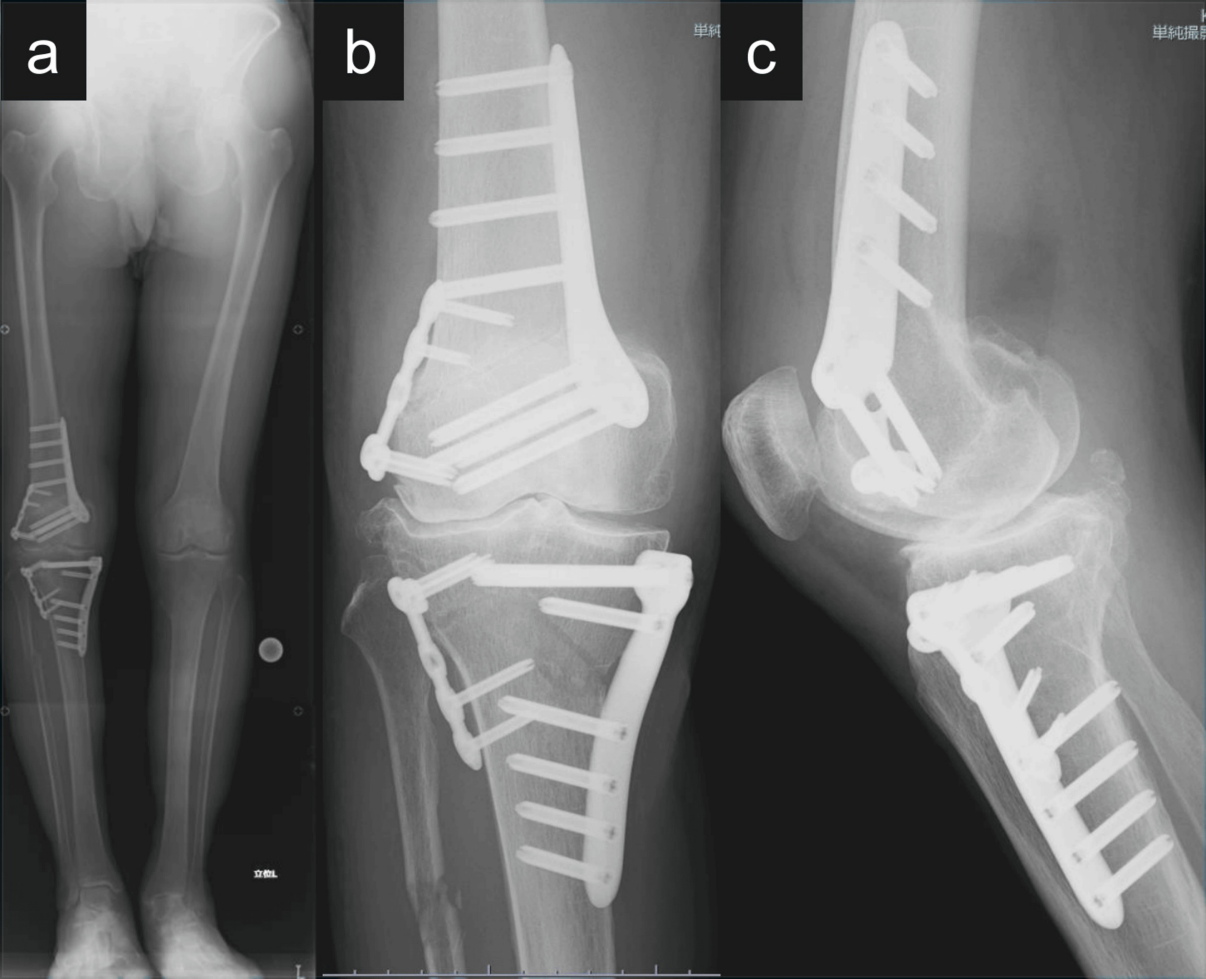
Immediate postoperative radiographs of the right knee following double-level osteotomy. (a) Standing full-length anteroposterior (AP) radiograph of the lower limbs demonstrating fixation after medial closing wedge distal femoral osteotomy and inverted V-shaped high tibial osteotomy. Internal fixation was achieved using the TriS Medial DFO Plate System (Olympus Terumo Biomaterial Corp., Tokyo, Japan) for the femoral side and the TriS Medial HTO Plate System (Olympus Terumo Biomaterial Corp.) for the tibial side. TriS Small Plates (Olympus Terumo Biomaterial Corp.) were additionally applied to the lateral aspects of both the femur and tibia to reinforce stability. (b) AP radiograph of the right knee displaying secure fixation of both osteotomy sites with clearly defined implant placement. (c) Lateral radiograph of the right knee showing well-aligned osteotomy lines and preservation of the posterior tibial slope.
